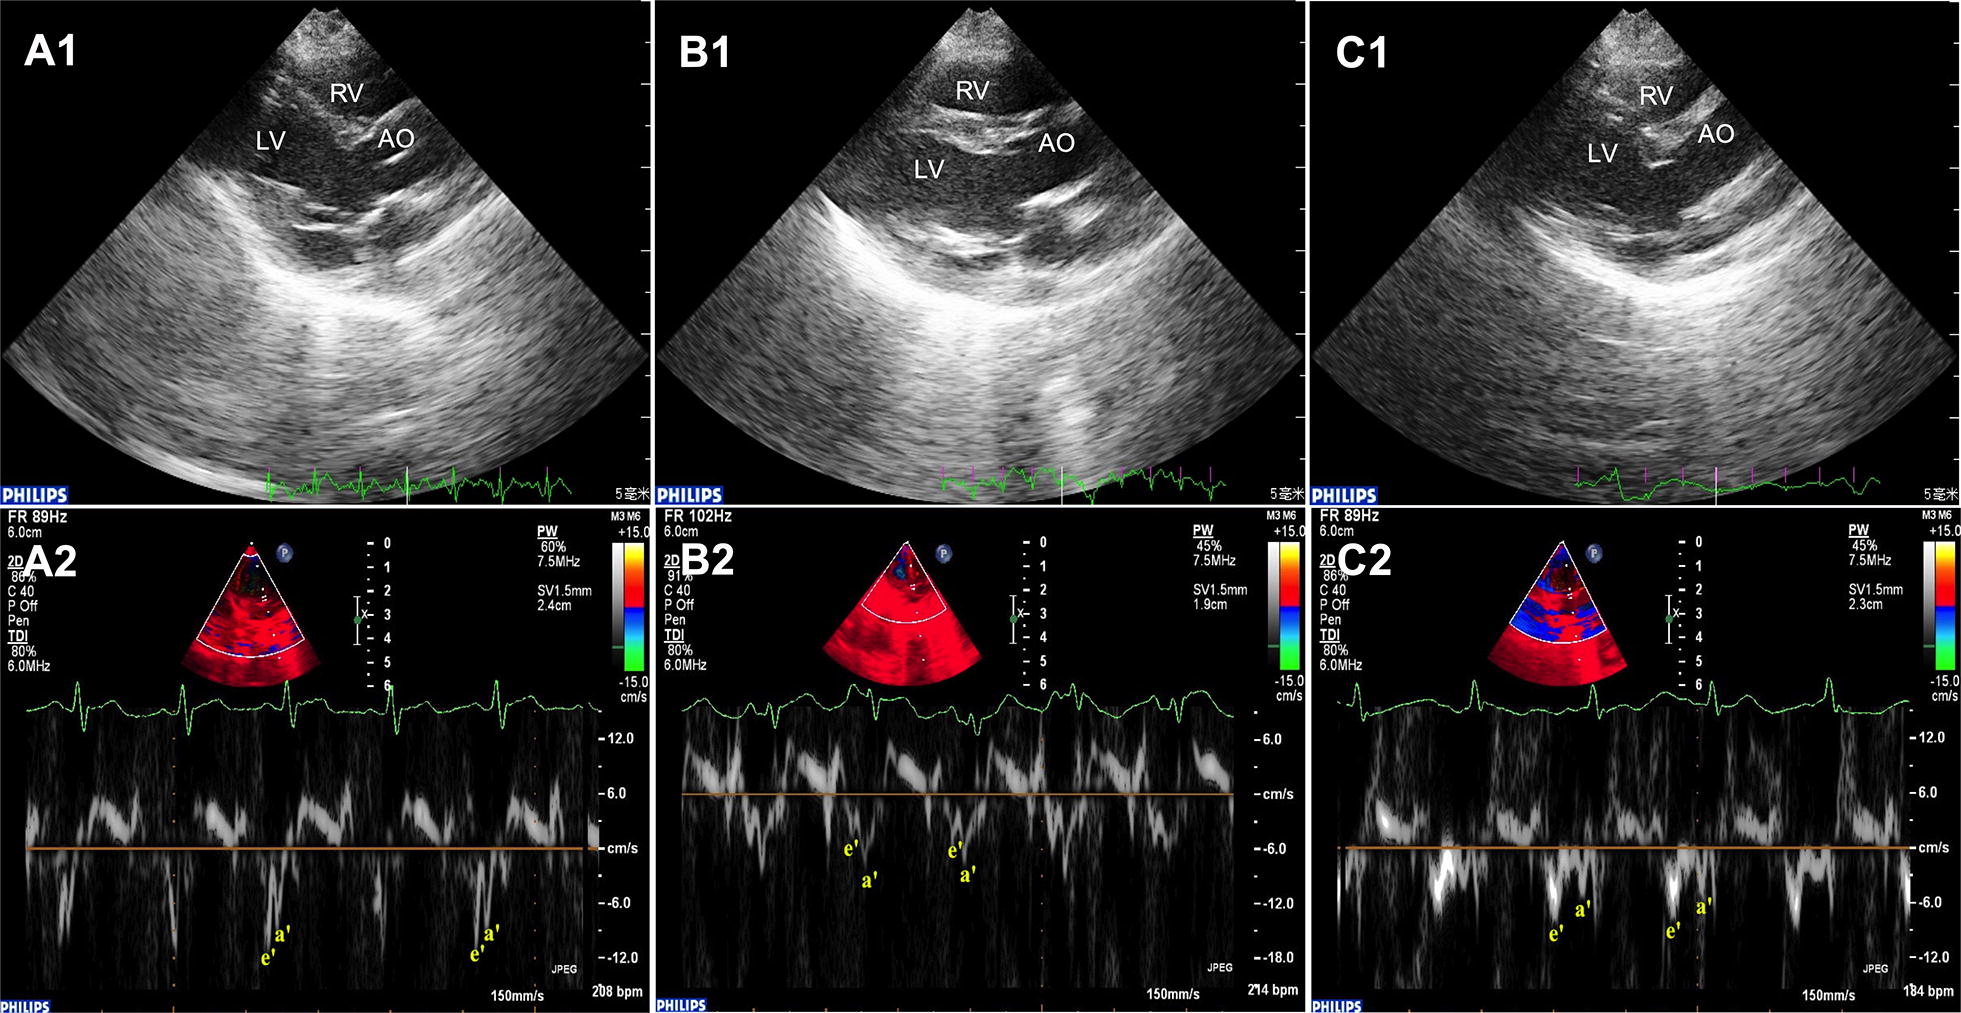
Representative echocardiographic images of the left ventricle for the three different groups. Two-dimensional echocardiographic image of the control group, diabetic group and alogliptin-treated diabetic group (A–C, top) and Doppler tissue imaging (bottom). *LV* left ventricle, *RV* right ventricle; *AO* aorta, *e′* early diastolic mitral annular velocity, *a′* late diastolic mitral annular velocity, n = 10 per group
